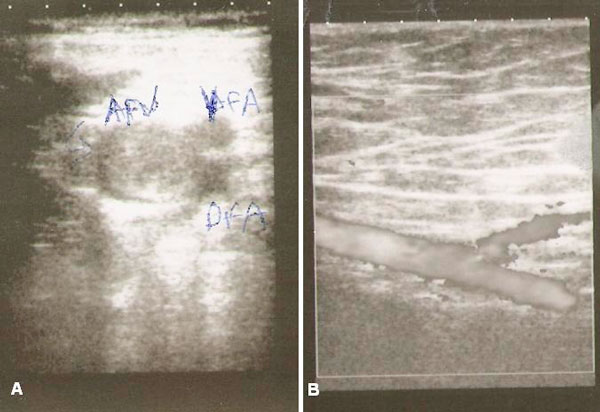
Doppler ultrasonography showing an acute phase thrombus without any response to augmentation. (B) Restored flow and disappearence of the thrombus after therapy.
